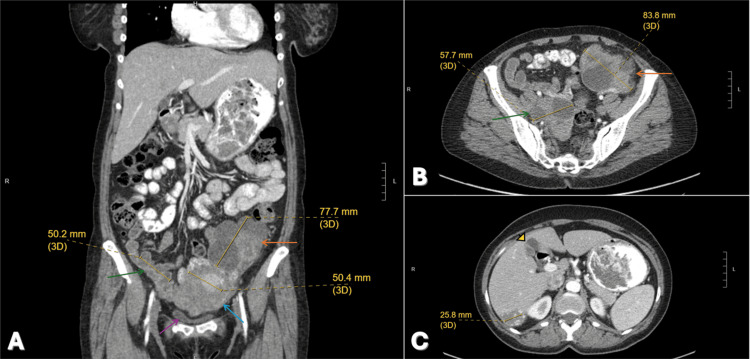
Coronal CT view of the patient A) Coronal CT view showing the uterus and ovaries are abnormal, with the uterus (blue arrow) containing multiple nodules and masses measuring up to 5.7 cm in diameter, with mass effects on the urinary bladder (purple arrow), bilateral adnexal masses (orange and green arrows), and free fluid (ascites) present in the pelvis. B) Axial CT view showing multiple simple cysts within the bilateral ovaries. The ovaries are enlarged, with the left measuring 9.8 x 6.6 cm in diameter (orange arrow) and the right 7.3 x 4.7 cm in diameter (green arrow). C) Axial CT view showing liver with two rounded hypodense nodules within the superior aspect of the right hepatic lobe, most likely cysts (arrowhead). There is a 3.5 cm right hepatic lobe hemangioma.
